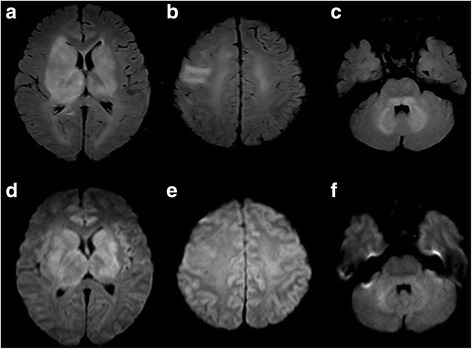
Nineteen-year-old man with lymphomatosis cerebri. a, b and c, Axial T2-weighted-FLAIR with STIR show the lesions distribution of type I, II and III respectively, which involved bilateral superior cerebellar peduncles, dentate nucleus of cerebellum, basal ganglia, internal capsule, thalamus and right frontal lobes. d, e and f, DWI images show slight hyperintensity in the lesions, but corresponding ADC maps (not shown) indicate no water of restriction
